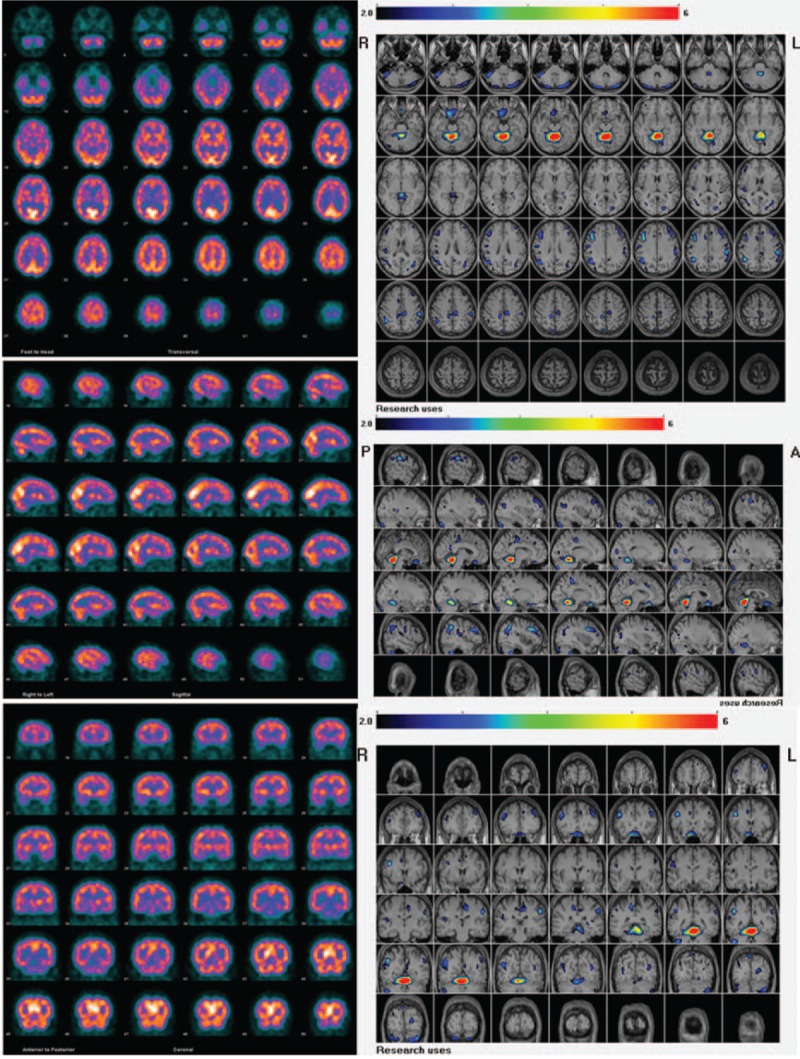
On conventional display of Tc-99m ECD brain perfusion single photon computed tomography (SPECT) (left column), no remarkable abnormality could be identified on SPECT images by readers of more than 5 experienced nuclear medicine physicians. The results of easy z-score imaging system analysis showed remarkable hypoperfusion at anterior lobe of cerebellum, in which red color on the fused magnetic resonance imaging template means Z-score higher than 6 (right column). In addition, mild grade of hypoperfusion in superficial areas of bilateral cerebellum, bilateral frontal, and parietal lobes (blue, green, and yellow colors means Z-score higher than 2 but less than 6).
